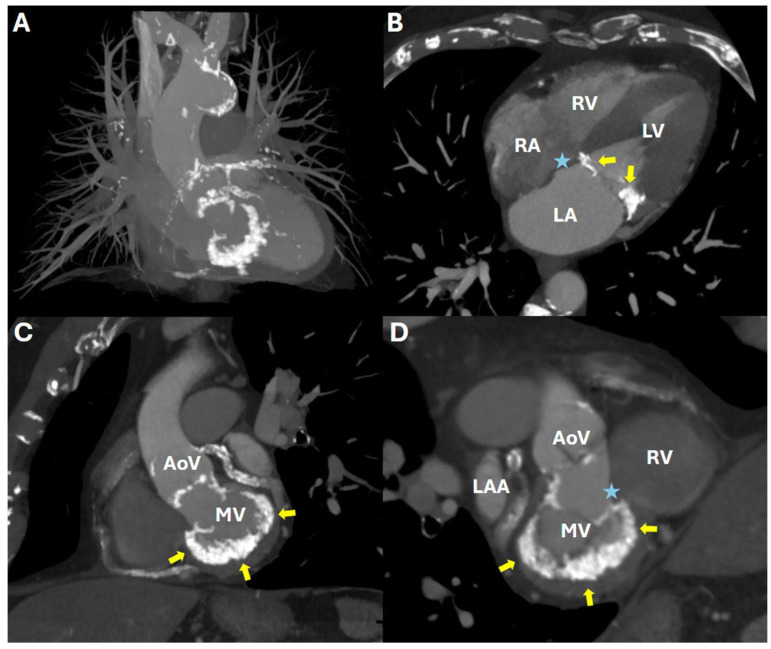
Cardiac computed tomography (CT) images of a patient with significant mitral annular calcification. Panel (A): three-dimenstional maximum intensity projection reconstruction overview of the heart and mediastinal great vessels. Panels (B,C): four-chamber and short axis double oblique reconstructions of the cardiac CT images and Panel (D): double oblique reformation of the heart, showing “*en face*” view of mitral annulus, demonstrating a more pronounced posterior and moderate anterior mitral annular calcification (yellow arrows) and the estimated location of the atrioventricular (AV) node (blue asterisks). AoV: aortic valve, LAA: left atrial appendage, LA: left atrium, LV: left ventricle, MV: mitral valve, RA: right atrium, RV: right ventricle.
